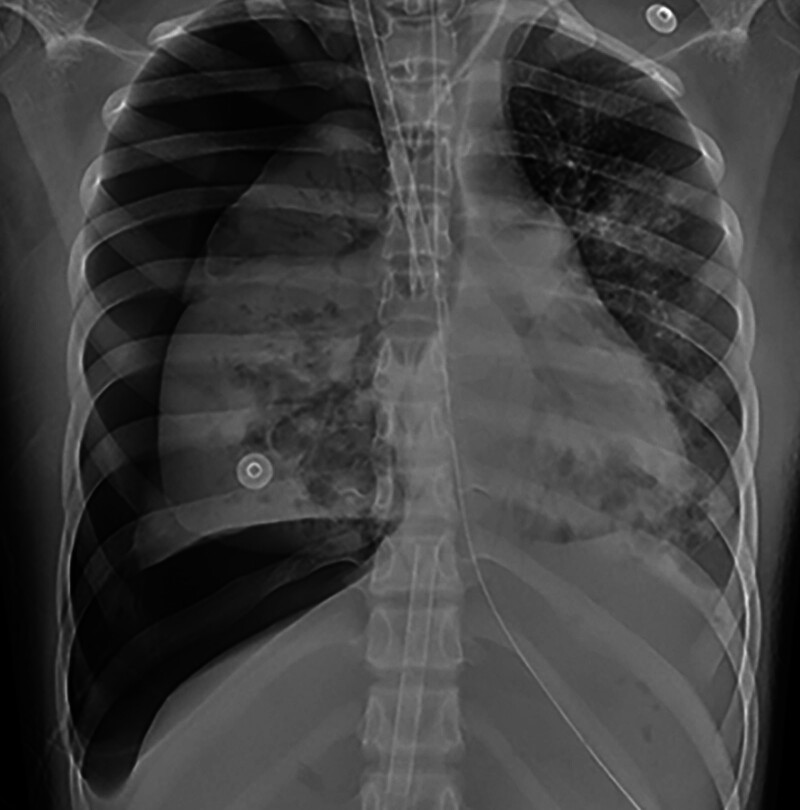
February 27, 2023 chest X-rays (CXRs) revealed right side liquid pneumothorax (gas about 60%). CXRs = chest X-rays.
